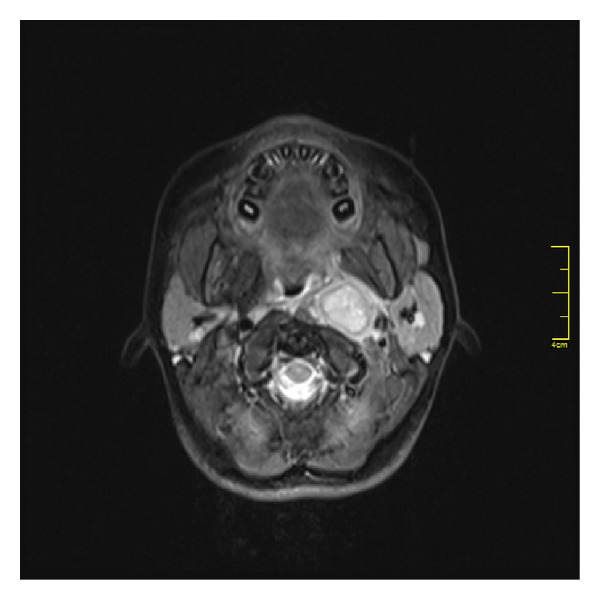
In the contrasted neck MRI, there was 3 × 3 cm axial size, hyperintense in T2 weighted, left parapharyngeal space occupying lesion on the left infratemporal fossa adjacent to the medial-inferior border.
